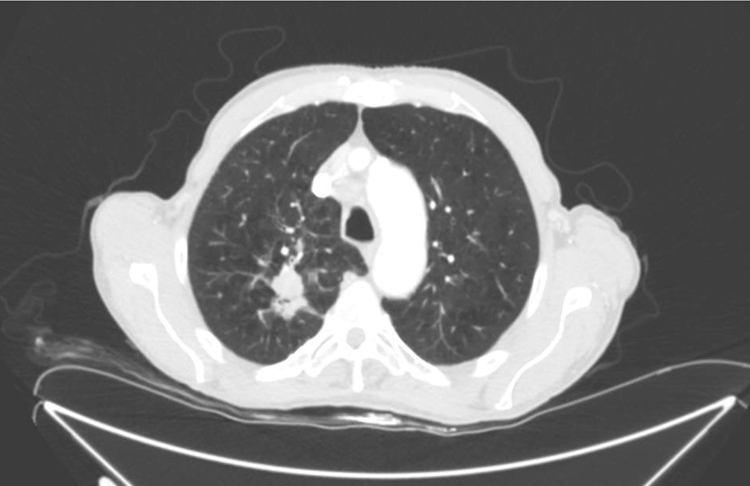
Computed tomography scan findings: 22 × 34 mm nodular lesion with spiculated borders on the upper right lobe with right supraclavicular, hilar, precaval and subcarinal lymphadenopathies.
